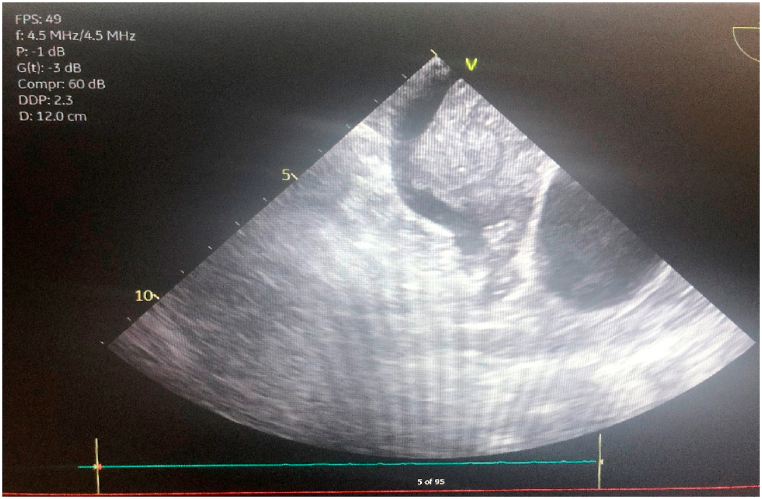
Left atrial mass seen on *trans*-esophageal echocardiogram in the OR.
